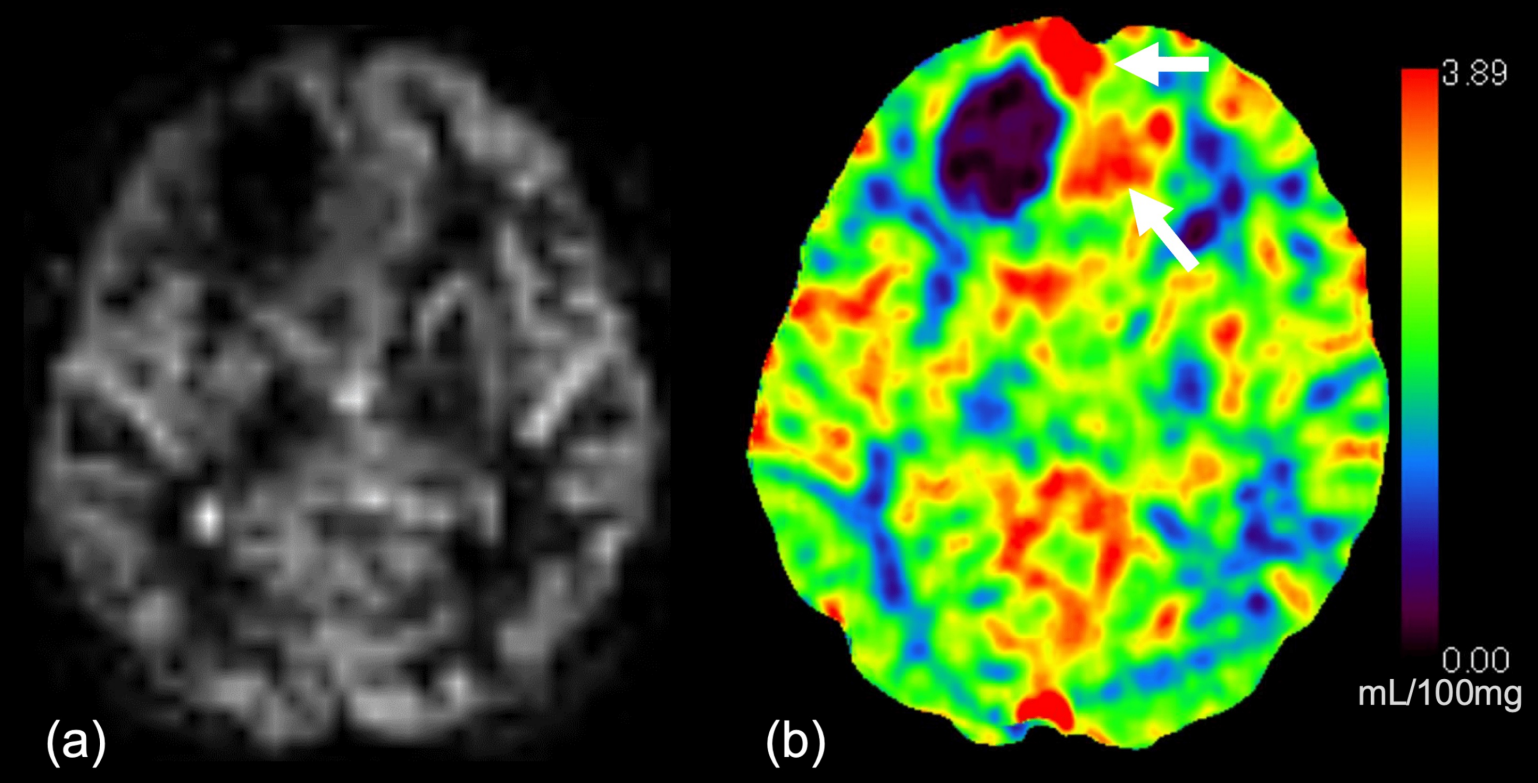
ASL and CTP images Pseudo-continuous ASL demonstrates no evidence of a cortical high-flow sign, a feature that has been reported in association with oligodendrogliomas, IDH-mutant and 1p/19q-codeleted (a). Cerebral blood volume from CTP mapping reveals increased perfusion in the medial portion of the tumor (b; arrows). ASL, Arterial spin labeling; CTP, Computed tomography perfusion
